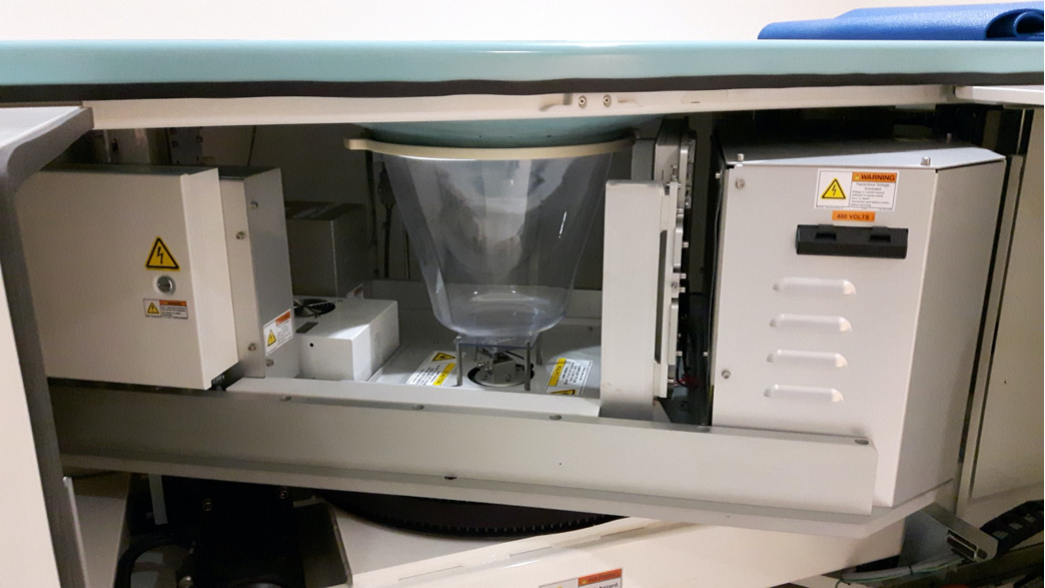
Photo of a flat panel cone beam dedicated breast CT system showing the main components of the imaging chain. The plastic cup located surrounding the space for the pendant breast isolates the moving parts of the system from the breast and the rest of the patient.
